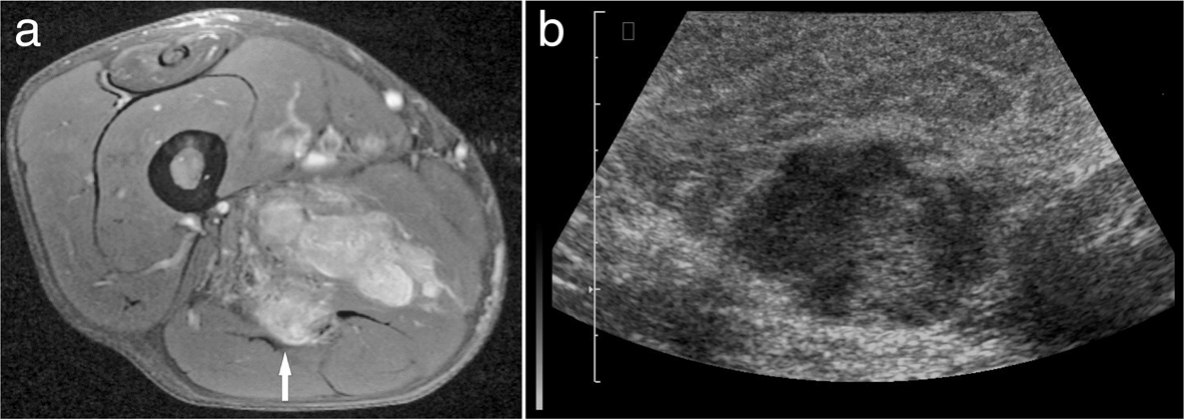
MM in the thigh musculature in a patient with known history of metastatic renal cell carcinoma. (a) T1w image (turbo spin echo sequence with fat saturation, TR/TE: 615/11 ms) after contrast administration shows a lobulated mass (arrow) with inhomogeneous enhancement (arrow). On ultrasound, the mass was mixed hypo- to hyperechoic in comparison to the unaffected muscle (b).
